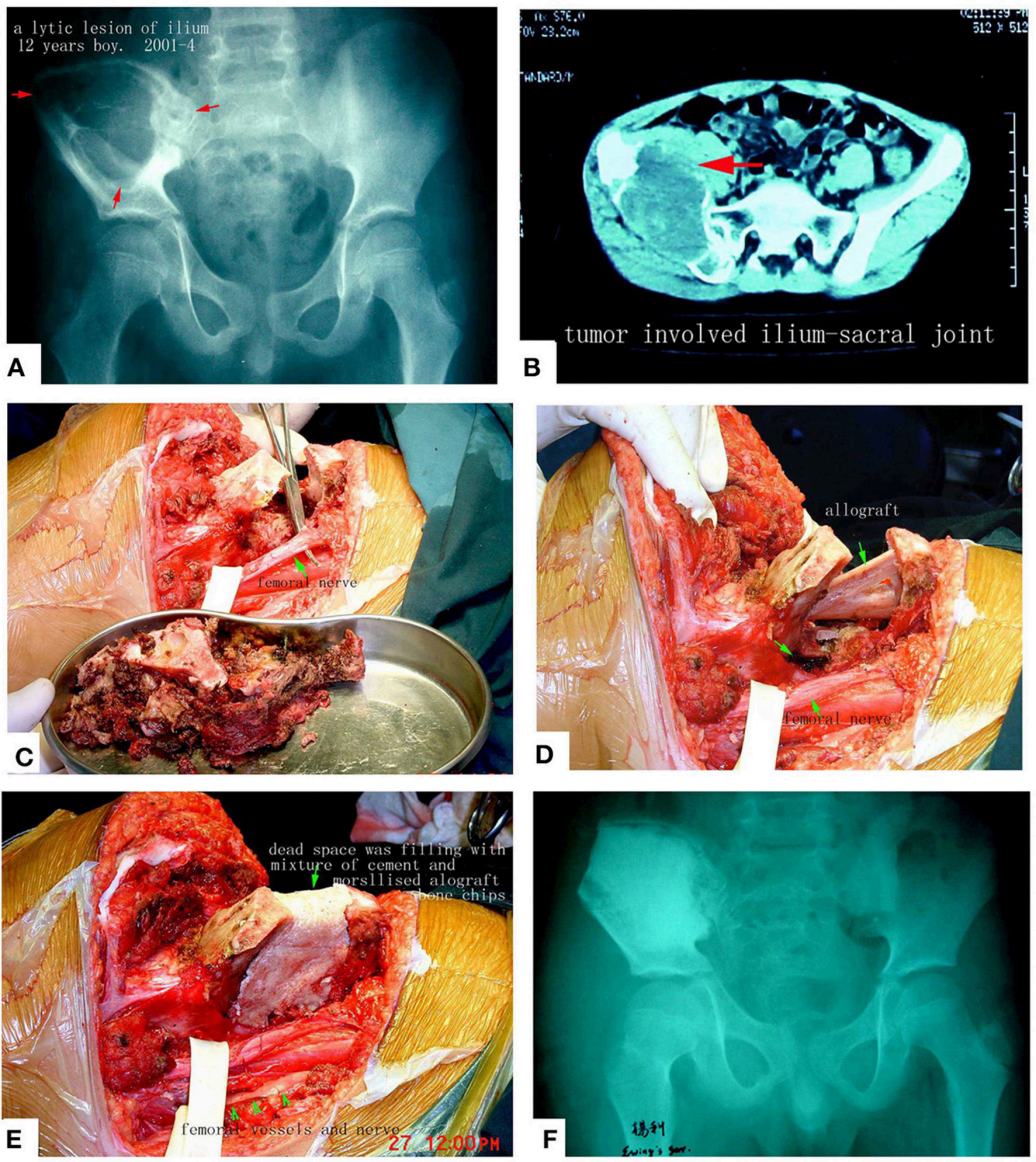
Pelvic Ewing's sarcoma treated by MWA. (A) X-ray film before surgery. (B) CT scan. (C) The soften, devitalized tumor tissues were removed. (D,E) Reconstruction by allograft and cement. (F) X-ray after the operation. The patient's function is perfect.
